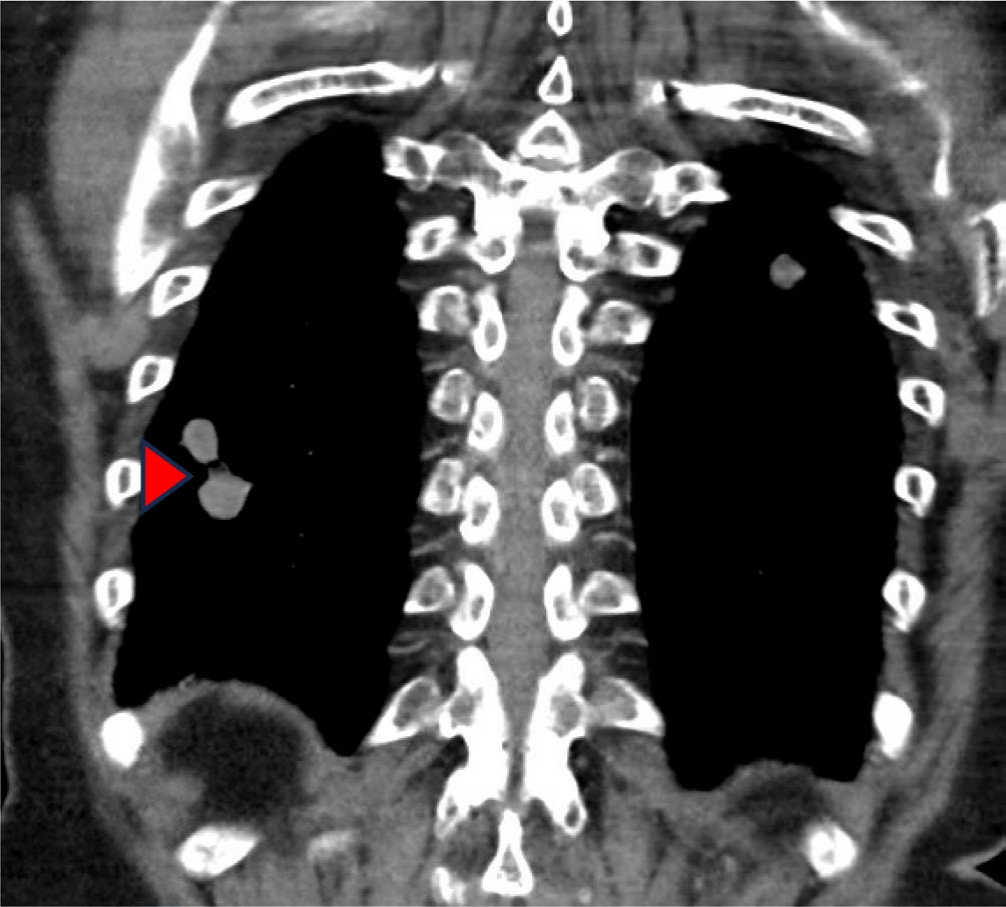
FDG PET‐CT scan showing pulmonary metastases along the oblique fissure in the right lobe (see arrow) prior to treatment with pembrolizumab and pazopanib.
